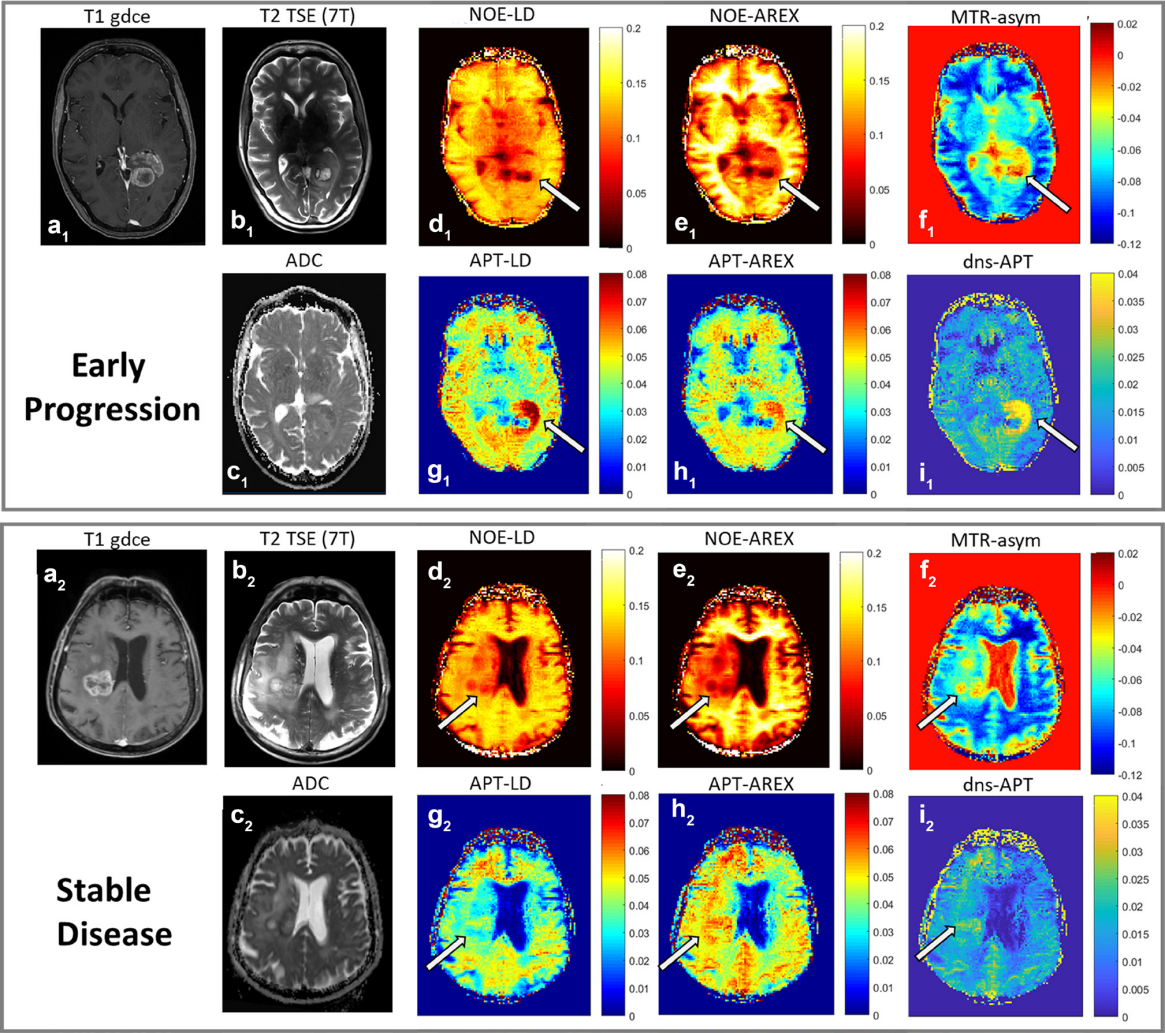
Exemplary CEST MR-images Top: Early progression. Bottom: Stable disease. a_1_ and a_2_: T1-weighting with gadolinium enhancement (3 Tesla), b_1_ and b_2_: T2-weighted TSE (7 Tesla), c_1_ and c_2_: ADC-map (3 Tesla), d_1_ and d_2_: NOE-LD, e_1_ and e_2_: NOE-AREX, f_1_ and f_2_: NOE-weighted MTR_asym_, g_1_ and g_2_: APT-LD, h_1_ and h_2_: APT-AREX, i_1_ and i_2_: dns-APT. NOE-LD and -AREX-mediated images show a decreased tumor signal, with much lower values in the early progression case (arrows in d_1_–e_1_ compared to d_2_–e_2_). The opposite is true for the MTR_asym_ contrasts (arrow in f_1_ compared to f_2_). APT-mediated images show a considerably higher signal intensity within the early-progressive tumor (arrows in g_1_–i_1_ compared to g_2_–i_2_).
